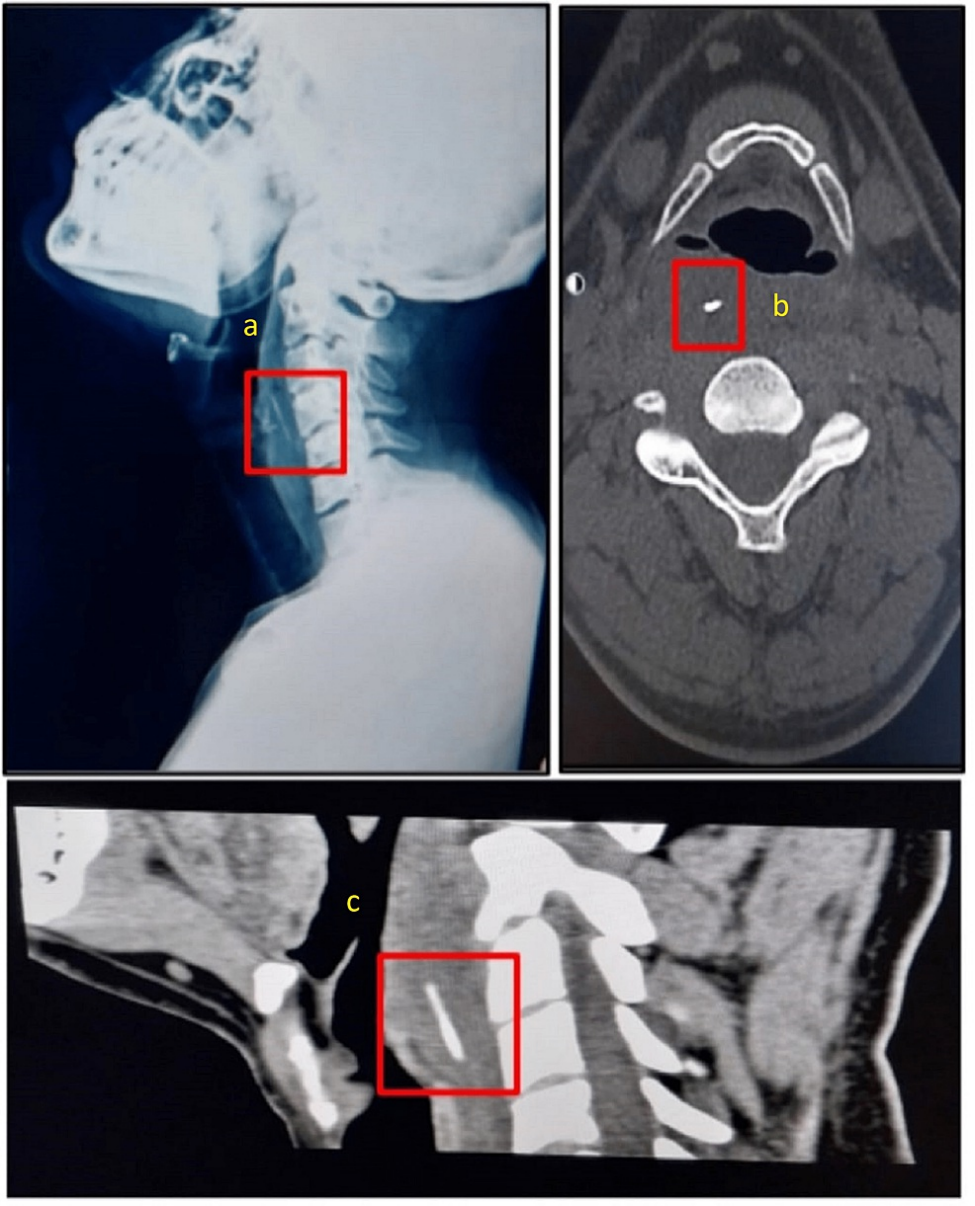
Radiological investigations suggestive of a foreign body (a) Plain X-ray of the head and neck (lateral view) showing a radio-opaque foreign body at the level of the hyoid bone. (b) Axial CT image showing the linear hyperdense structure at the level of the hyoid bone, likely a foreign body. (c) Sagittal reformatted plain CT showing a 2.1 cm linear hyperdense structure extending obliquely and posteromedially from hyoid to apex of pyriform fossa, 1 cm lateral to the midline, and 1 cm in depth from the posterior pharyngeal wall at C2 vertebral level, likely a foreign body.
